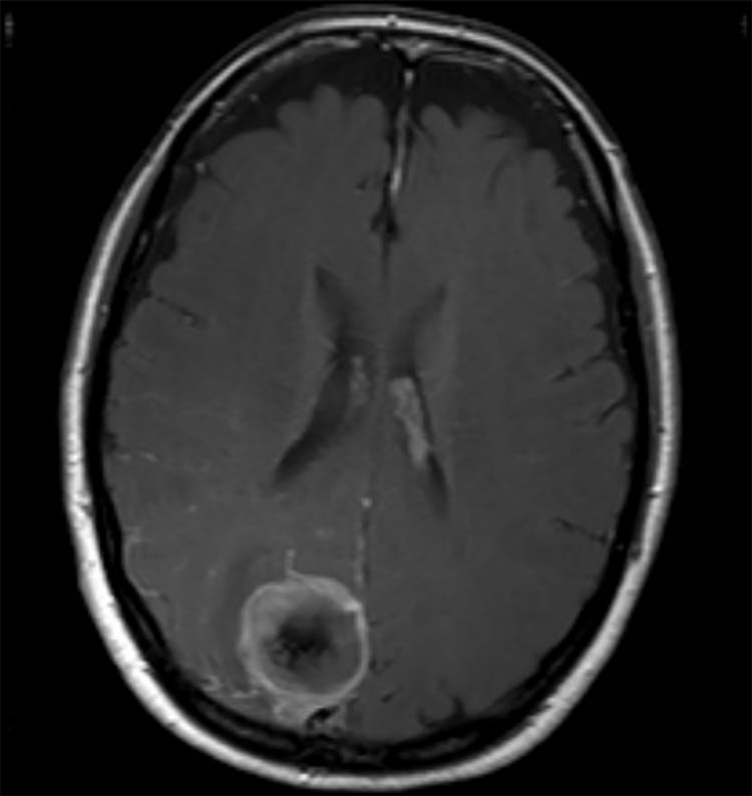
Axial view of a T1 weighted MRI post-gadolinium enhancement demonstrating the appearance of a 3-cm right parieto-occipital extra-axial mass with bony involvement and adjacent small nodule. Most in keeping with a meningioma.
